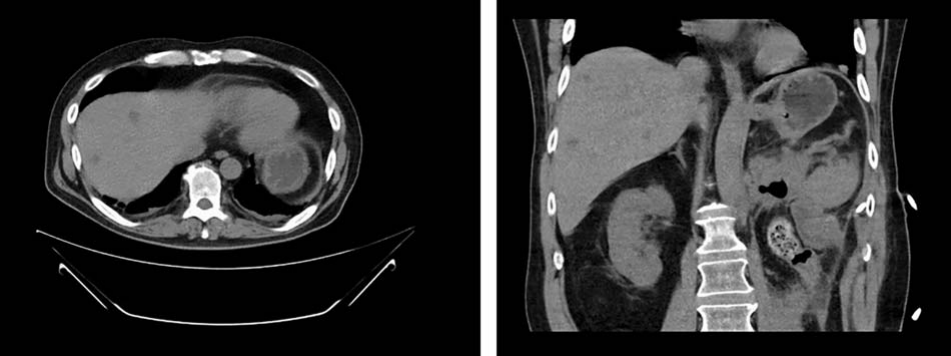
First appearance of multiple liver lesions suspicious for metastasis in transverse (left) and coronal (right) planes on CT; reduction of retroperitoneal effusion can also be seen.
